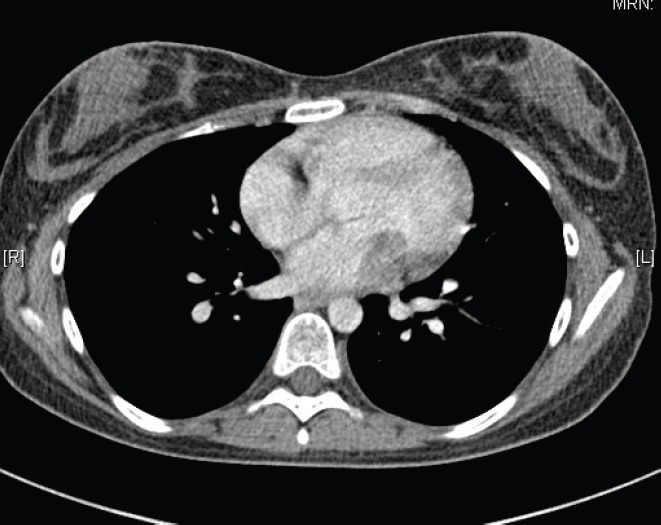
2013 CT thorax with contrast.
